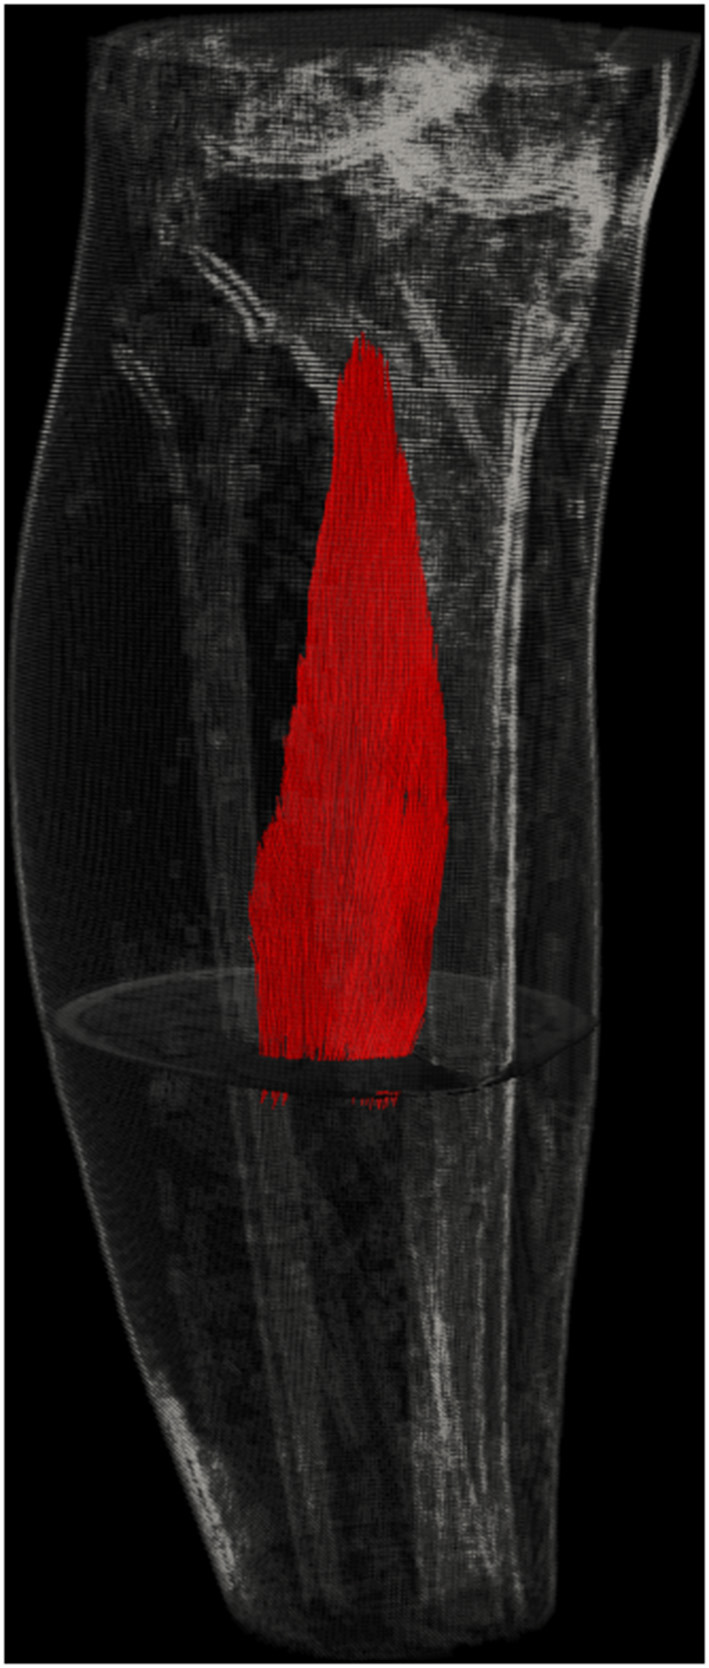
Visualization of human muscle using MRI and DTI. Utilization of advanced imaging techniques offers major potential for analysis of structure as well as local muscle length and shape changes for human muscles *in vivo*.
